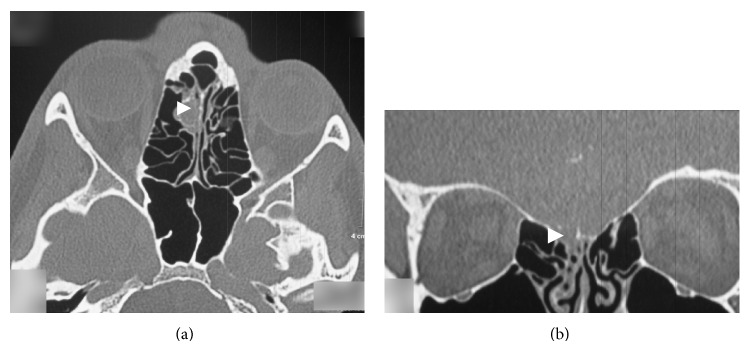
((a)-(b)) CT scan for patient with suspected CSF leakage. Defect in anterior ethmoidal cells in lamina cribrosa area.
